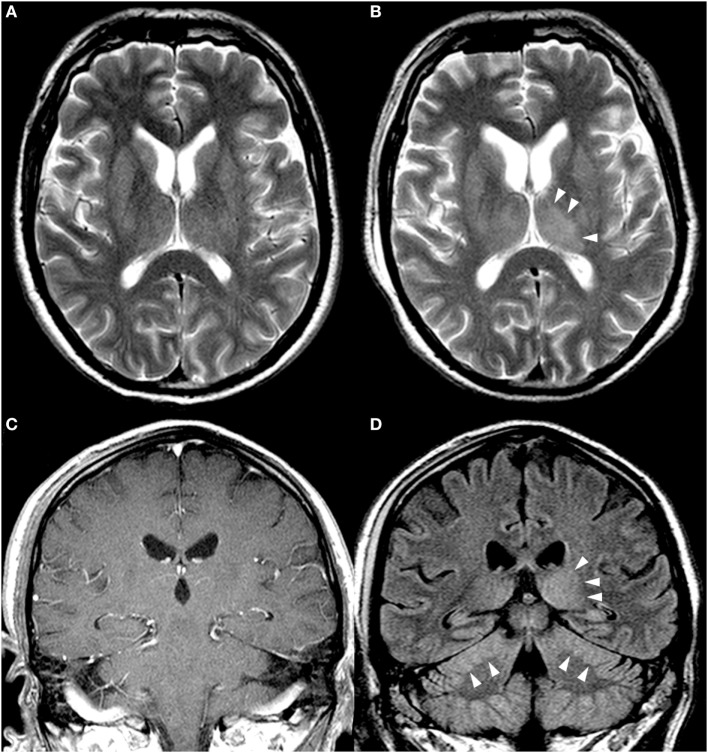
Cerebral magnetic resonance imaging (MRI): (A,B) T2-weighted axial scans. (C) T1-weighted coronal scan after intravenous application of gadolinium contrast medium. (D) Coronal scan with fluid attenuated inversion recovery imaging (FLAIR). (A) MRI on day 3. (B–D) MRI on day 16, showing an asymmetry with a minimal increase of signal intensity in the left thalamus (B,D) and in the cerebellum, with slight swelling and compression of the cerebellar sulci (D). No pathological contrast enhancement was seen (C).
